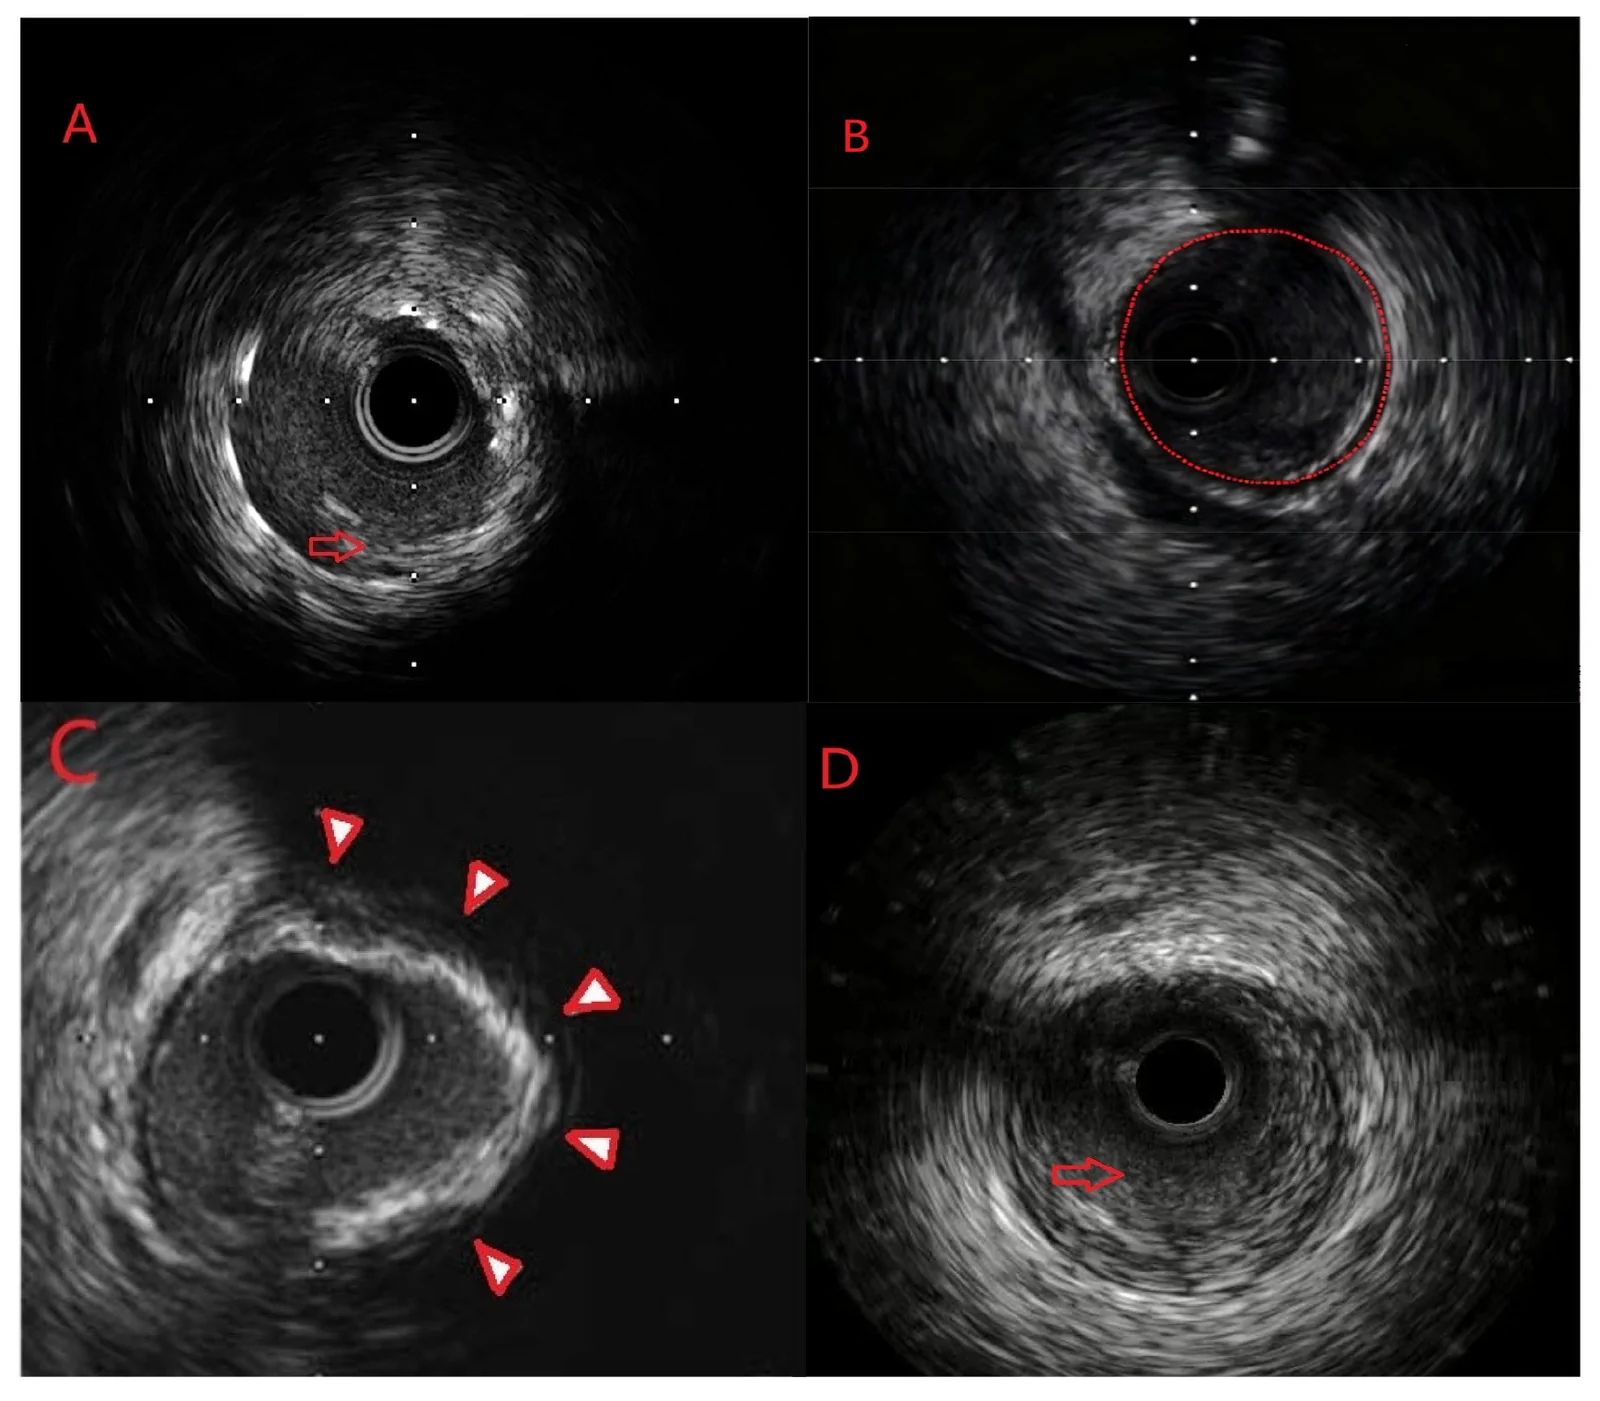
Lesion characteristics in IVUS to predict coronary no-reflow/slow-flow phenomenon. (A) Plaque prolapse: the protrusion of intra-stent tissue through stent struts (indicated by red arrow). (B) EEM cross-sectional area (outlined by red circle). (C) Calcification arc (indicated by red triangle). (D) Lipid plaque (indicated by red arrow). IVUS, intravascular ultrasound. EEM, external elastic membrane.
